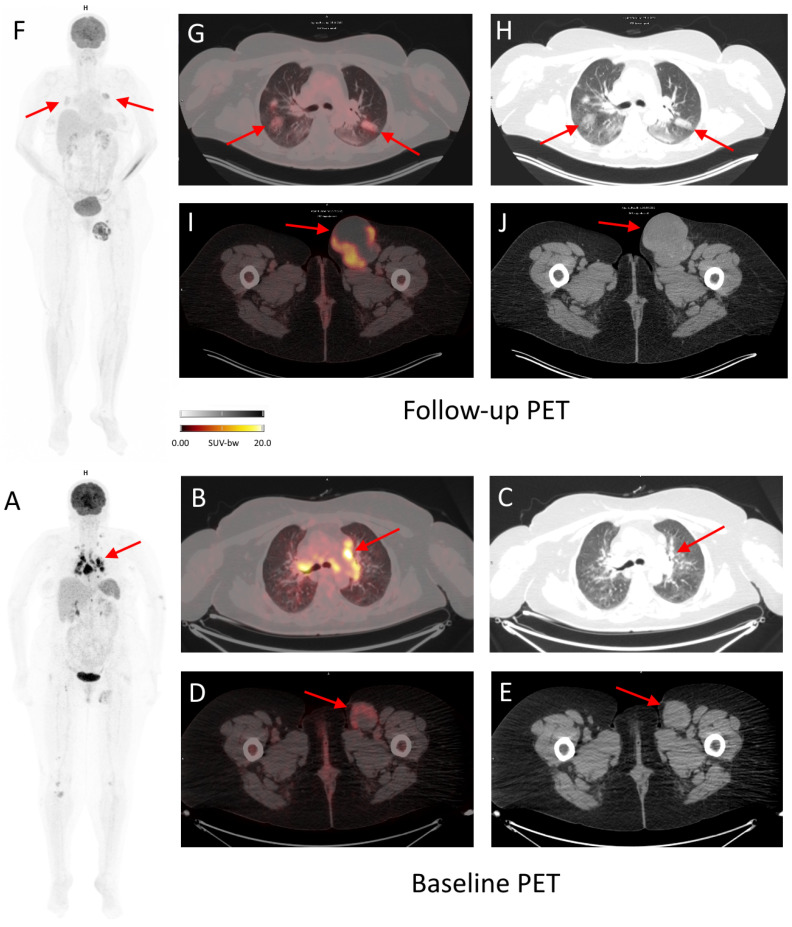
Baseline and follow-up ^18^F-FDG PET/CT of a 52-year-old woman with inoperable, stage IV melanoma treated with cemiplimab for 7 months. During the course of the treatment, the patient was diagnosed with immunotherapy-related pneumonia, grade 2, seen as metabolically active (SUVmax 7.3) ground glass opacity changes in both lungs ((F–H), arrows). The patient began treatment with prednisone (50 mg/day) with good radiographic response. The follow-up PET scan also revealed complete metabolic response of majority of pathological lymph nodes (seen in baseline PET, e.g., mediastinal, (A–C) arrows), except for the left inguinal lymph nodes, where progression was observed ((I,J) in follow-up PET vs. (D,E) in baseline PET; arrows), which could suggest dissociated response. However, due to previous immunotherapy with nivolumab and ipilimumab (before baseline PET), pathological lymph nodes in mediastinum should be differentiated between metastatic and due to sarcoid-like reaction.
